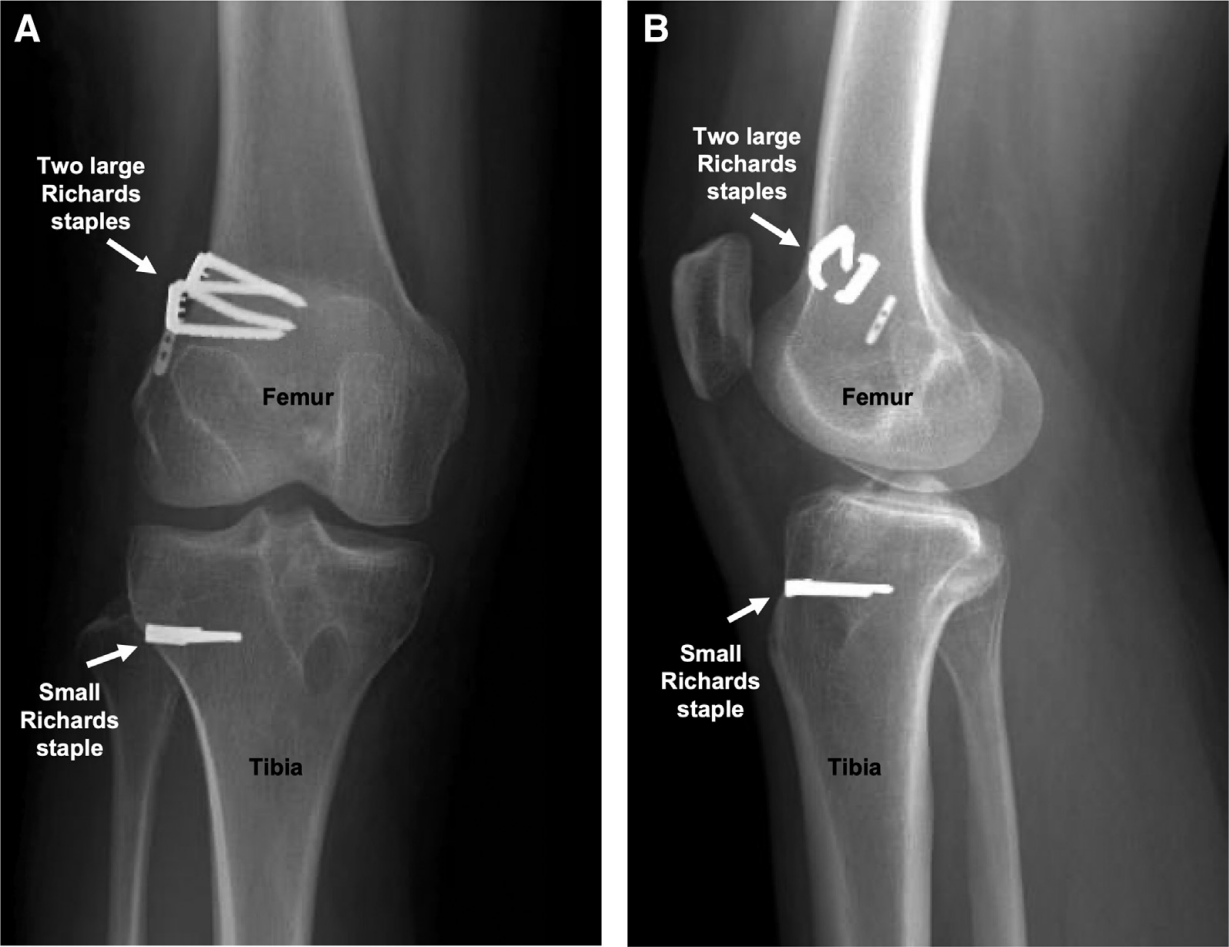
Femoral and tibial fixation of the Achilles tendon allograft using Richards staples (arrows) is verified on anteroposterior (A) and lateral (B) right knee radiographs.
